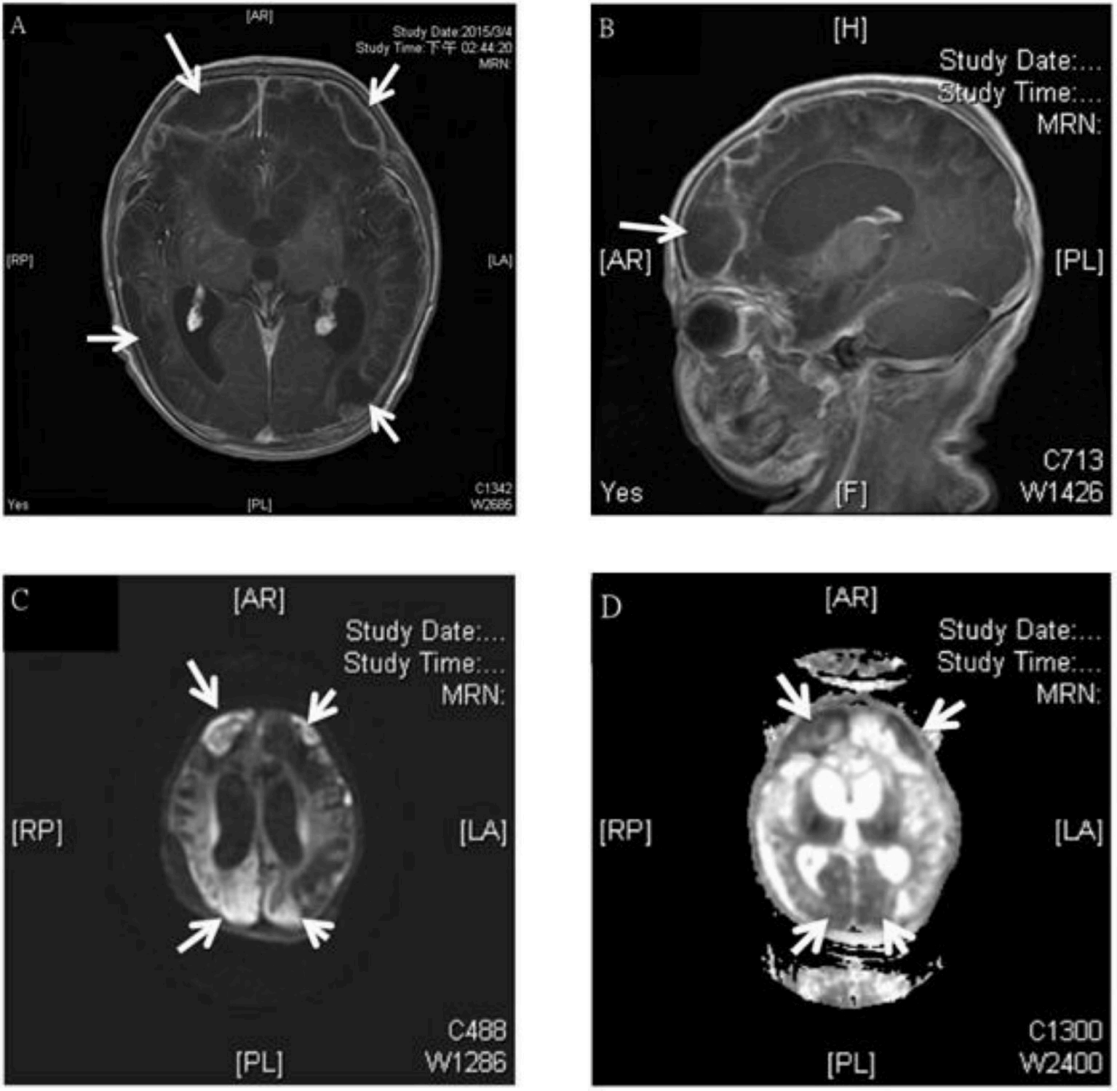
MRI study of a 3 week old boy with GBS subdural empyema. Axial (A) and sagittal (B) T1-weighted gadolinium-enhanced MRI showed subdural empyema over bilateral frontal, parietal, occipital area (arrows); diffusion-weighted (C), and apparent diffusion coefficient (D)-weighted MRI of a subdural empyema over bilateral frontal, parietal, occipital area (arrows).
